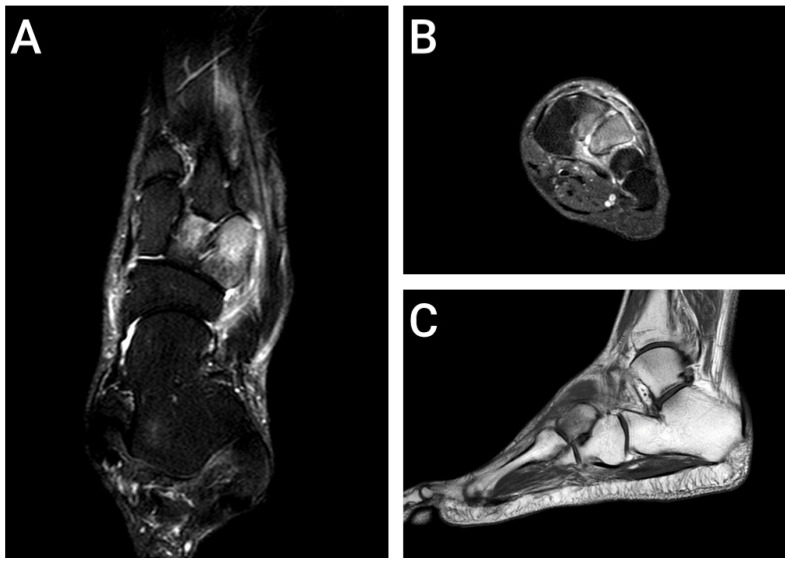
Bone marrow edema of the second and third cuneiform bones (midfoot), with mild periskeletal soft-tissue involvement, seen as hyperintensity on fat-saturated T2-weighted images in the axial (A) and coronal (B) planes and mild hypointensity on the sagittal T1-weighted image (C).
